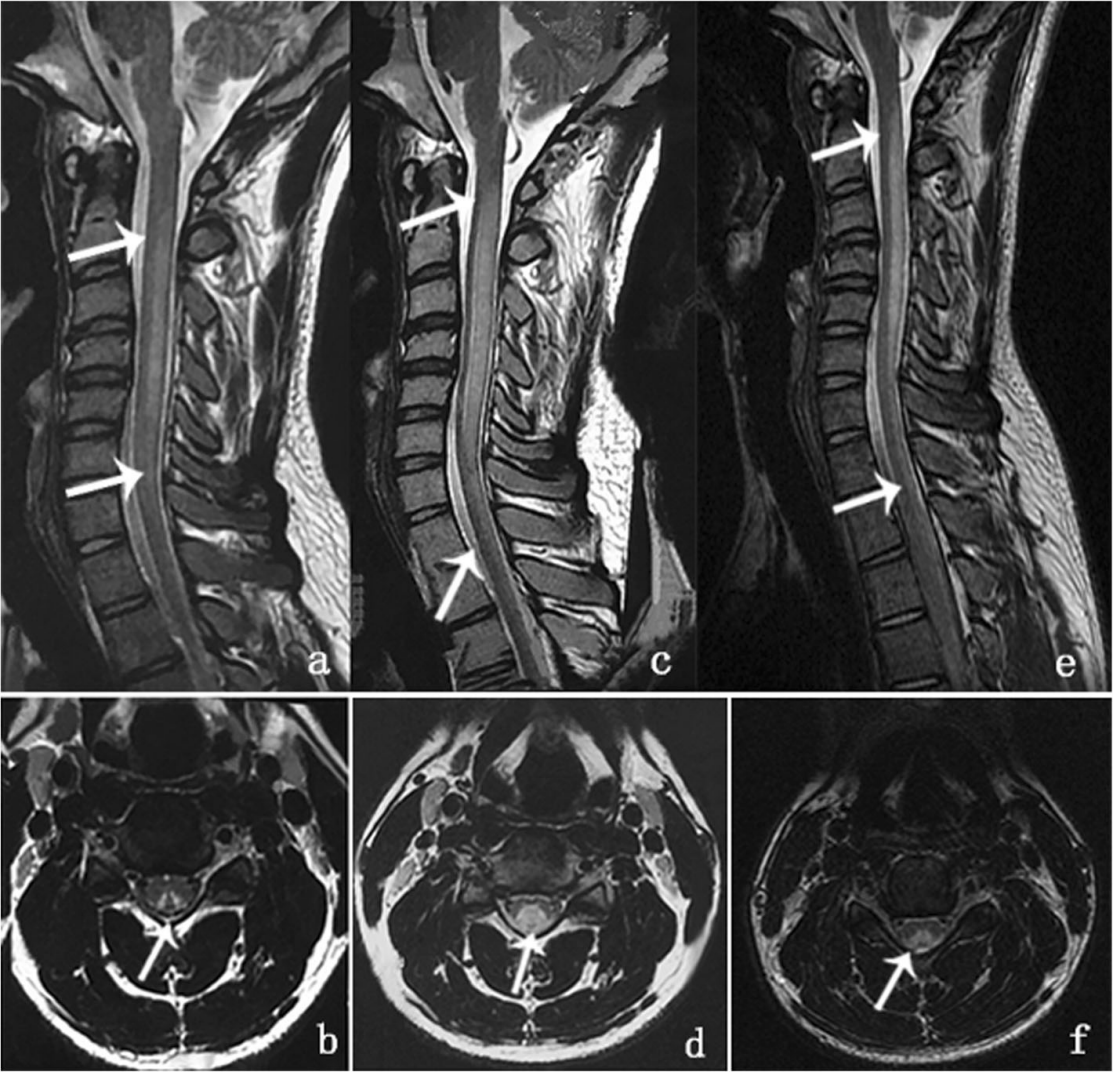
Magnetic resonance imaging of the cervical spinal cord. a. Sagittal T2-weighted imaging showed increased intramedullary signal intensity along the posterior column of the spinal cord extending from C2 to C6 on August 3, 2018. b. A V-shaped hyperintensity on axial T2-weighted imaging at the C4 level was seen within the dorsal cervical spinal cord on August 3, 2018. c. Sagittal T2-weighted imaging showed abnormal, longitudinally and horizontally extensive hyperintensities involving the lateral and posterior columns of the spinal cord extending from C1 through T2 on September 3, 2018. d. Axial T2-weighted imaging at the C4 level showed a ball-shaped hyperintensity on September 3, 2018. e. Sagittal T2-weighted imaging showed hyperintensity along the posterior column of the spinal cord extending from C1 to T1 on October 4, 2018. f. Axial T2-weighted imaging at the C4 level showed that the V-shaped hyperintensity had decreased compared to that in September (d)
